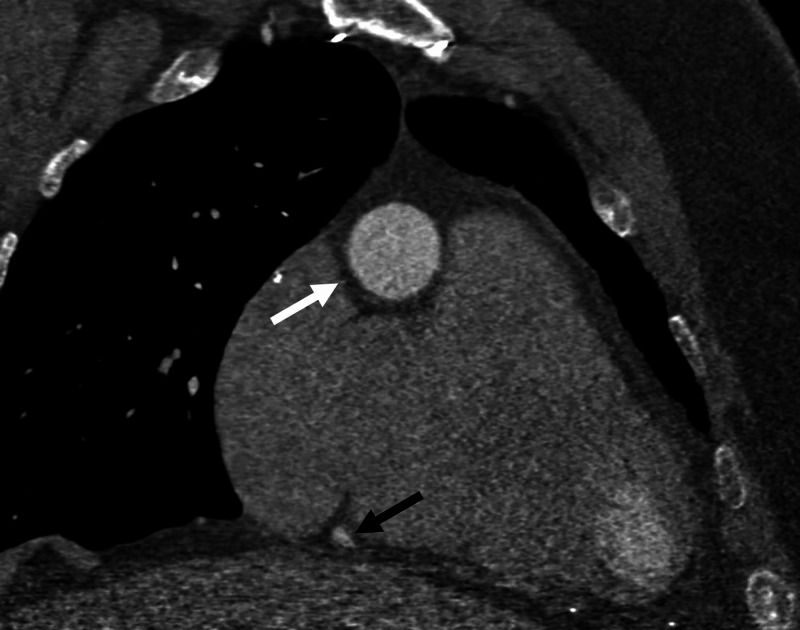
Multiplanar Reconstruction Oblique Coronal CTA Image Right coronary artery demonstrates aneurysmal dilatation of the artery just distal to the ostium (white arrow). The caliber of the distal right coronary artery is normal (black arrow).
